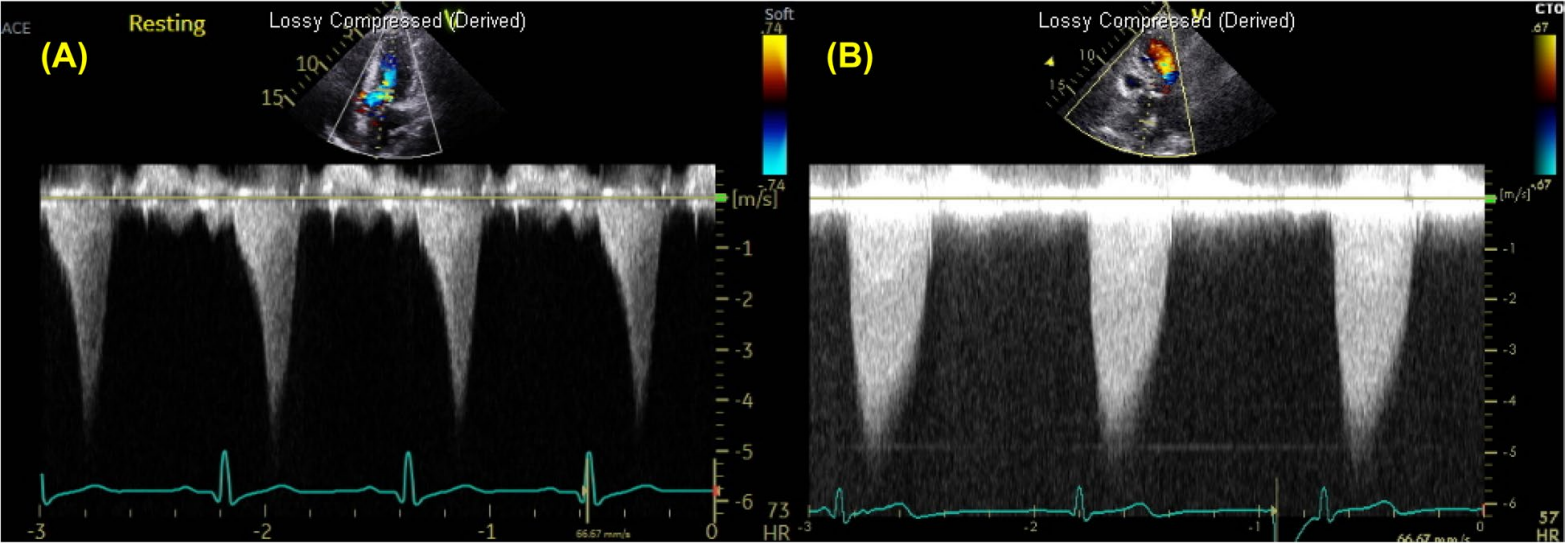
Continuous-wave Doppler demonstrates (A) the characteristic “dagger-shaped” profile in a patient with hypertrophic cardiomyopathy and dynamic left outflow tract obstruction, compared to (B) the relatively rounded profile seen in aortic stenosis
